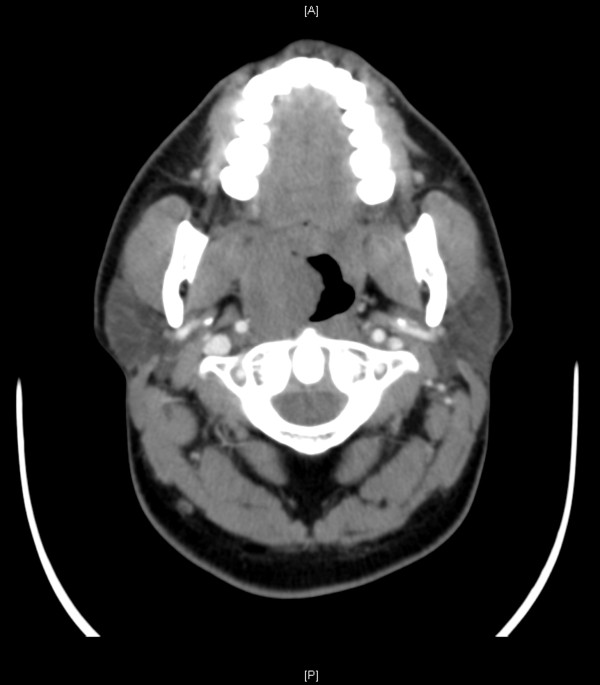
Iodinated, contrast-enhanced computed tomography scan of the head and neck (cross-section) showing non-enhancing, homogenous enlargement of the right tonsil.
